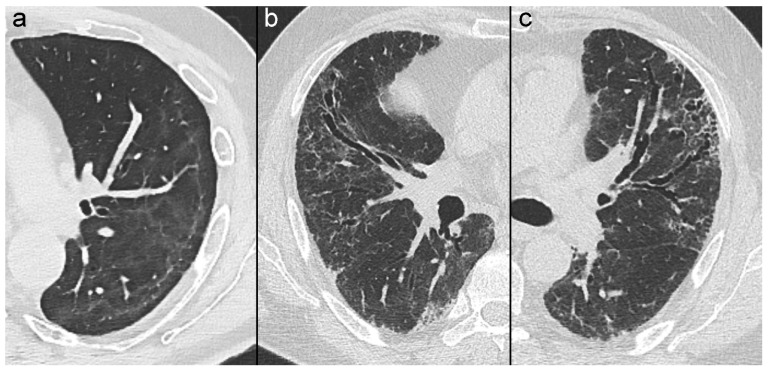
Cropped axial thin-section computed tomography (CT) images with lung window setting show some examples of long-term chest CT findings in survivors of coronavirus disease (COVID-19): (a) ground-glass and subpleural curvilinear opacities; (b) reticular opacities with architectural distortion and bronchiectasis; (c) reticular opacities with architectural distortion and traction bronchiectasis.
